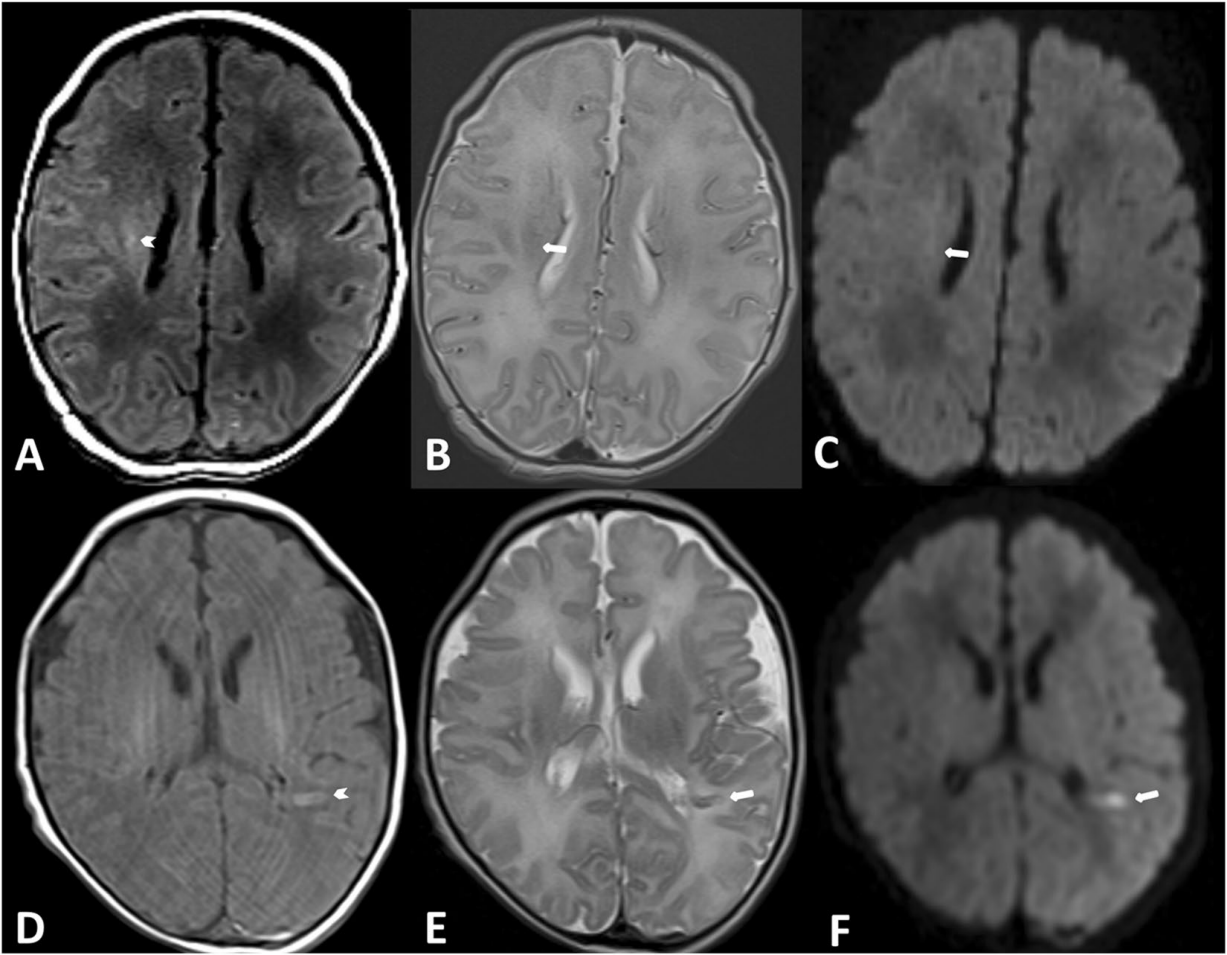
Examples of a WMI (upper row) and a stroke (lower row). A WMI is seen in the right semioval centre with T1w hyperintense signal intensity and no signal changes in T2w and DWI (patient No. 8). An acute stroke is seen in the white matter on the left, with signal changes in T1w and T2w and diffusion restriction in the DWI (patient No. 3).
